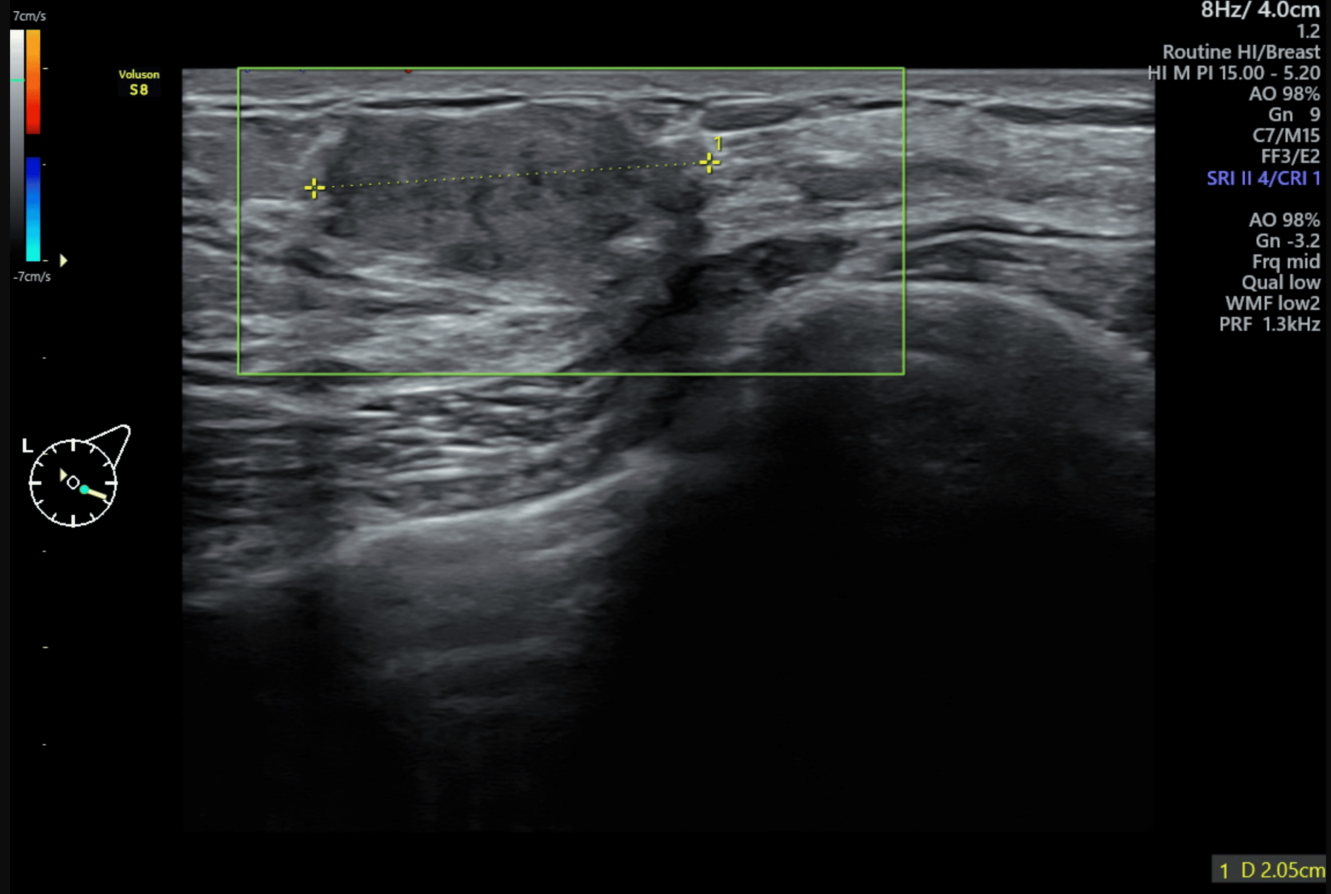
Ultrasound appearance of the adenomyoepithelioma A well-circumscribed hypoechoic lesion with a maximum diameter of 2.1 cm, lacking vascularity, and oriented parallel to the skin was noted.
